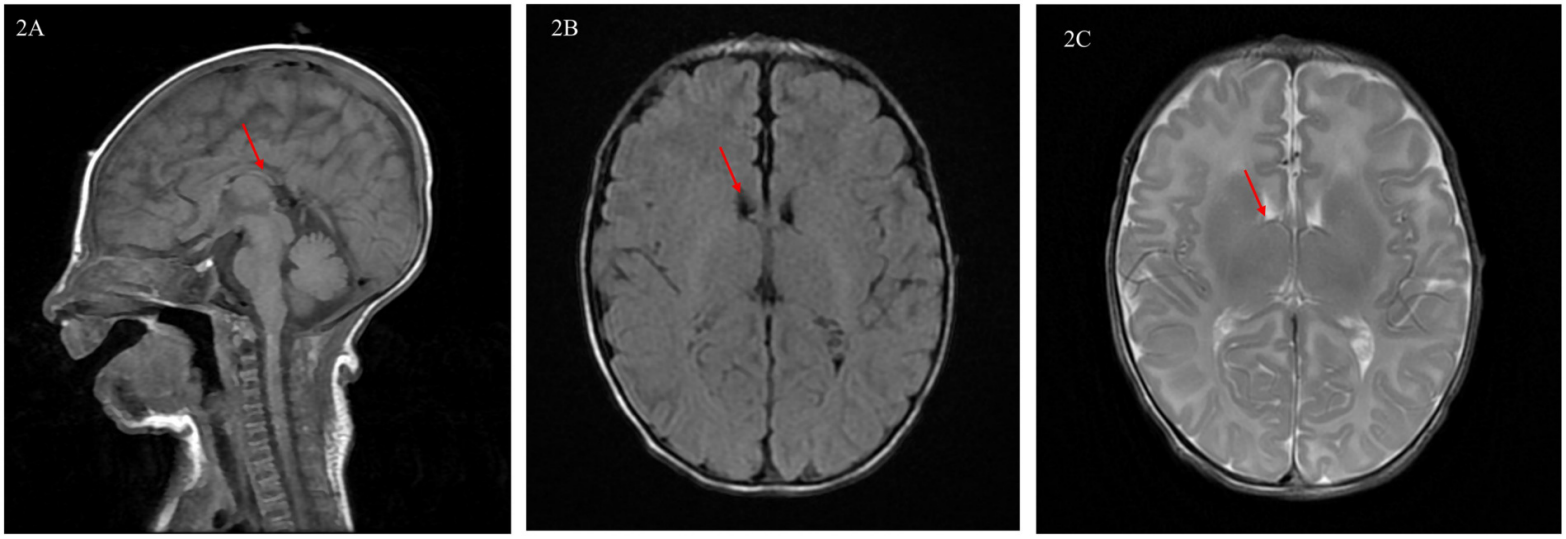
Brain MRI of a newborn with SSR4-CDG (onset in the neonatal period). (A) T1-weighted sagittal images suggest: Short corpus callosum (GE-3.0T). (B) T2-FLAIR axial images demonstrate mild contour irregularity of the bilateral lateral ventricles. (C) Axial T2-weighted images demonstrate mild irregularity in the contour of both lateral ventricles (as indicated by the arrows).
